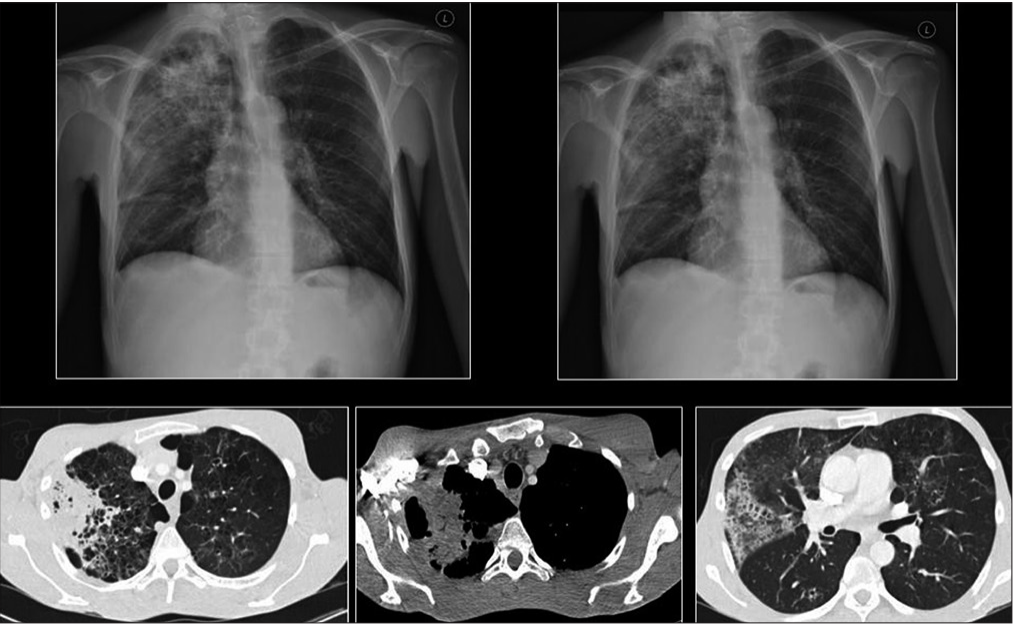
Mycobacterium xenopi* in a human immunodeficiency virus, 36-year old, male patient with a CD4 count of 80 with four positive sputum samples and a bronchoalveolar lavage for acid-fast bacilli. The chest X-ray and computed tomography scans show cavitating consolidation, loss of volume, traction bronchiectasis and ground-glass appearances in the right apical region superimposed on bullous disease of the lungs. There is no associated lymphadenopathy
